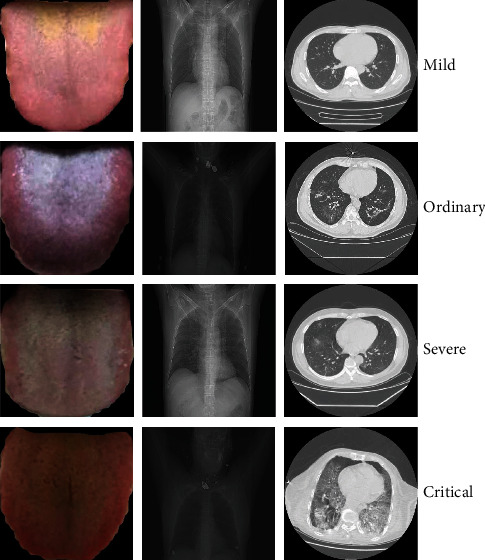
Samples of different types of modalities: tongue image, chest CT, and chest X-rays.
